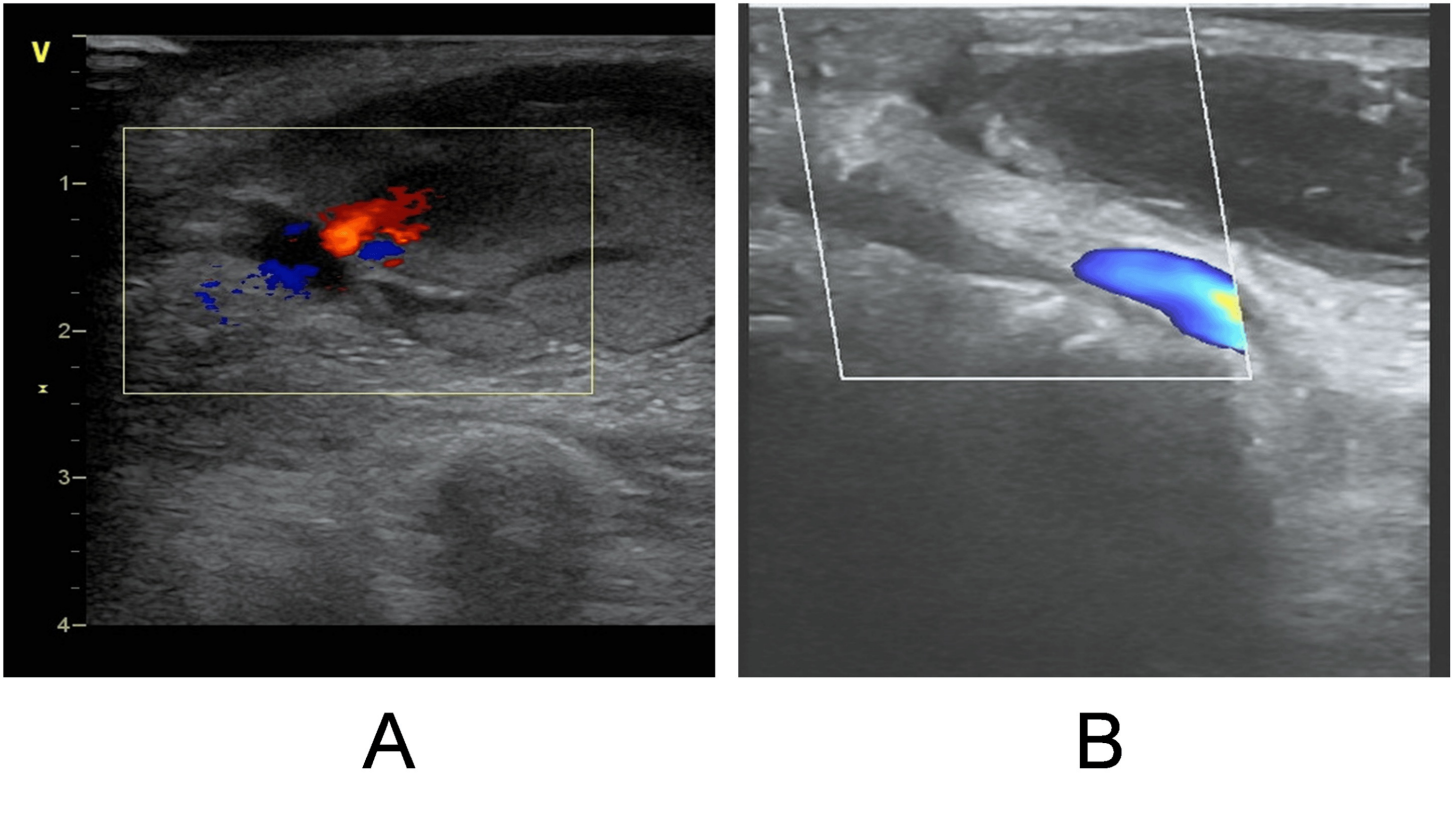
Pseudoaneurysm under ultrasound A. pseudoaneurysm before compression dressing with laceration; B. after pressure bandaging the pseudoaneurysm, the rupture disappeared.
